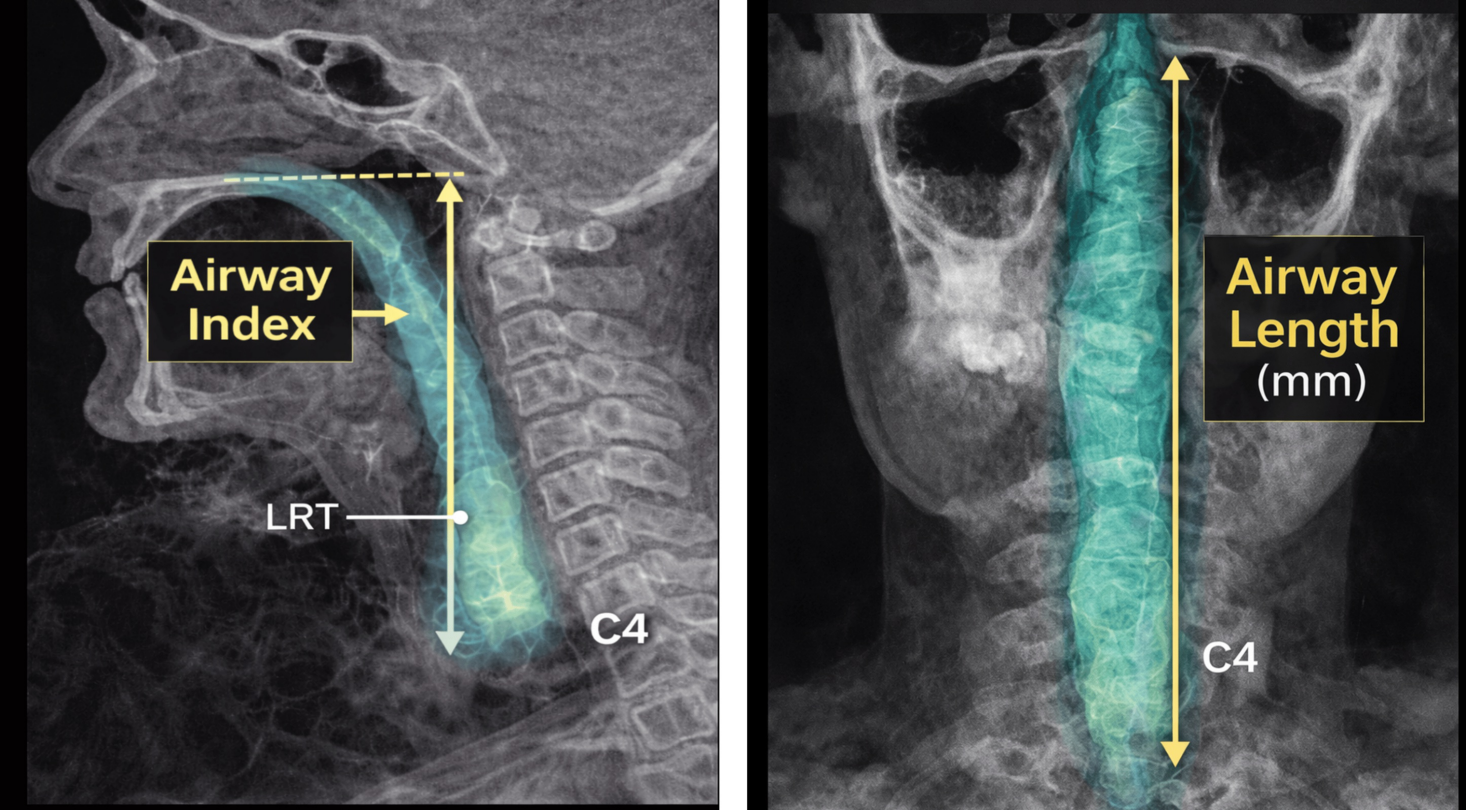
CBCT depiction of airway length and airway index measurement landmarks CBCT: Cone-beam computed tomography
